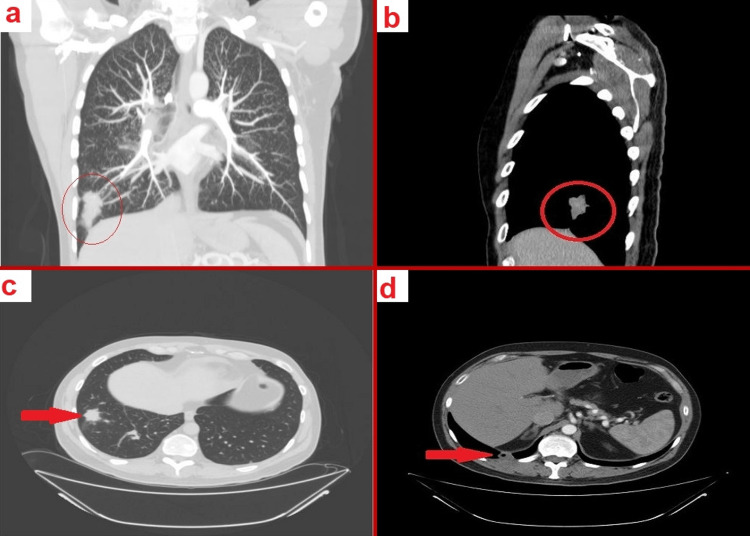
CT scan of the chest, abdomen, and pelvis with IV contrast. a-d: Revealing lung lesions, and tiny centrilobular lung nodules, suggesting possible miliary metastasis.
